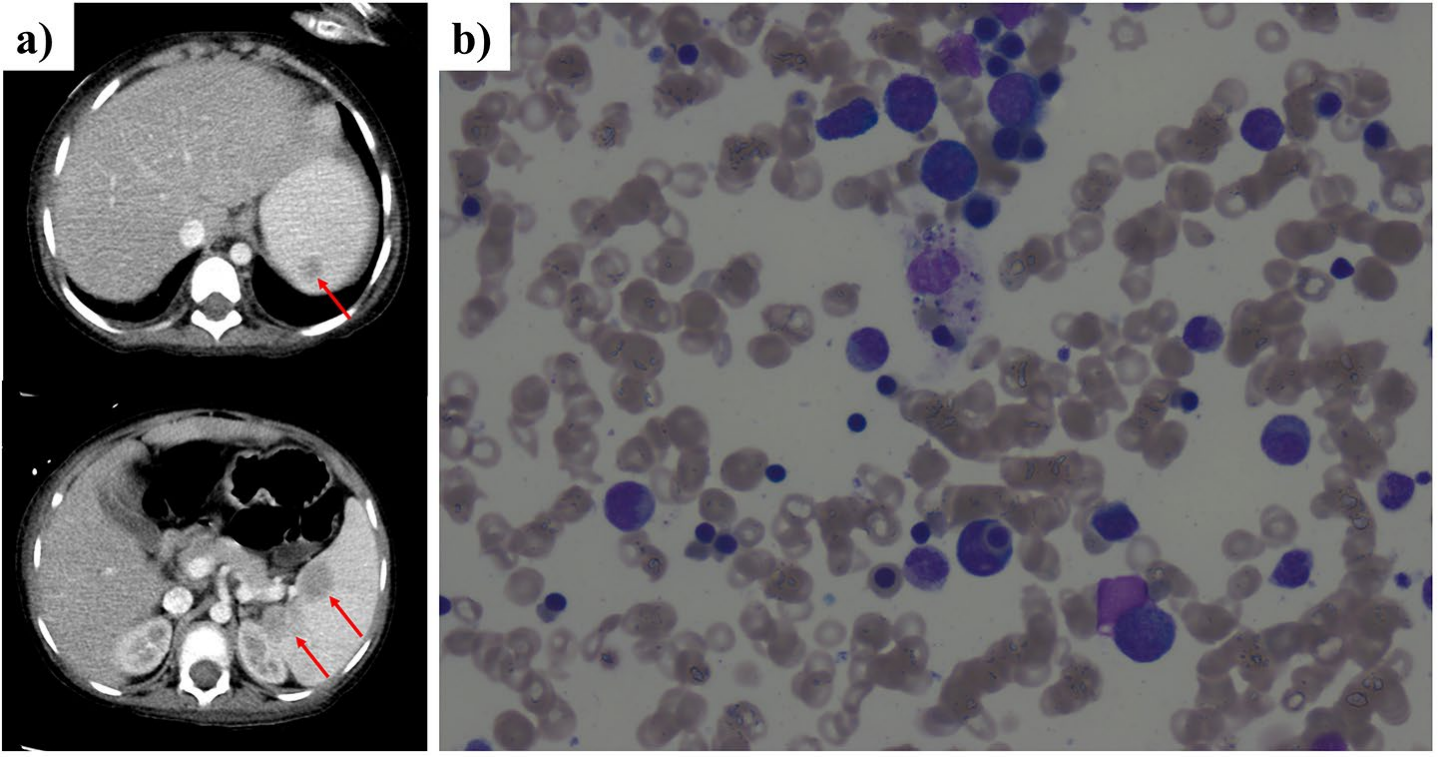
Abdomen CT and bone marrow aspirate findings. (a) Abdomen CT scan showed enlarged liver and spleen with multiple abscesses (red arrows). (b) BMA showing hemophagocytic activity with macrophages engulfing mainly neutrophils, red blood cells, and platelets. No increased blasts or lymphocytes are observed
